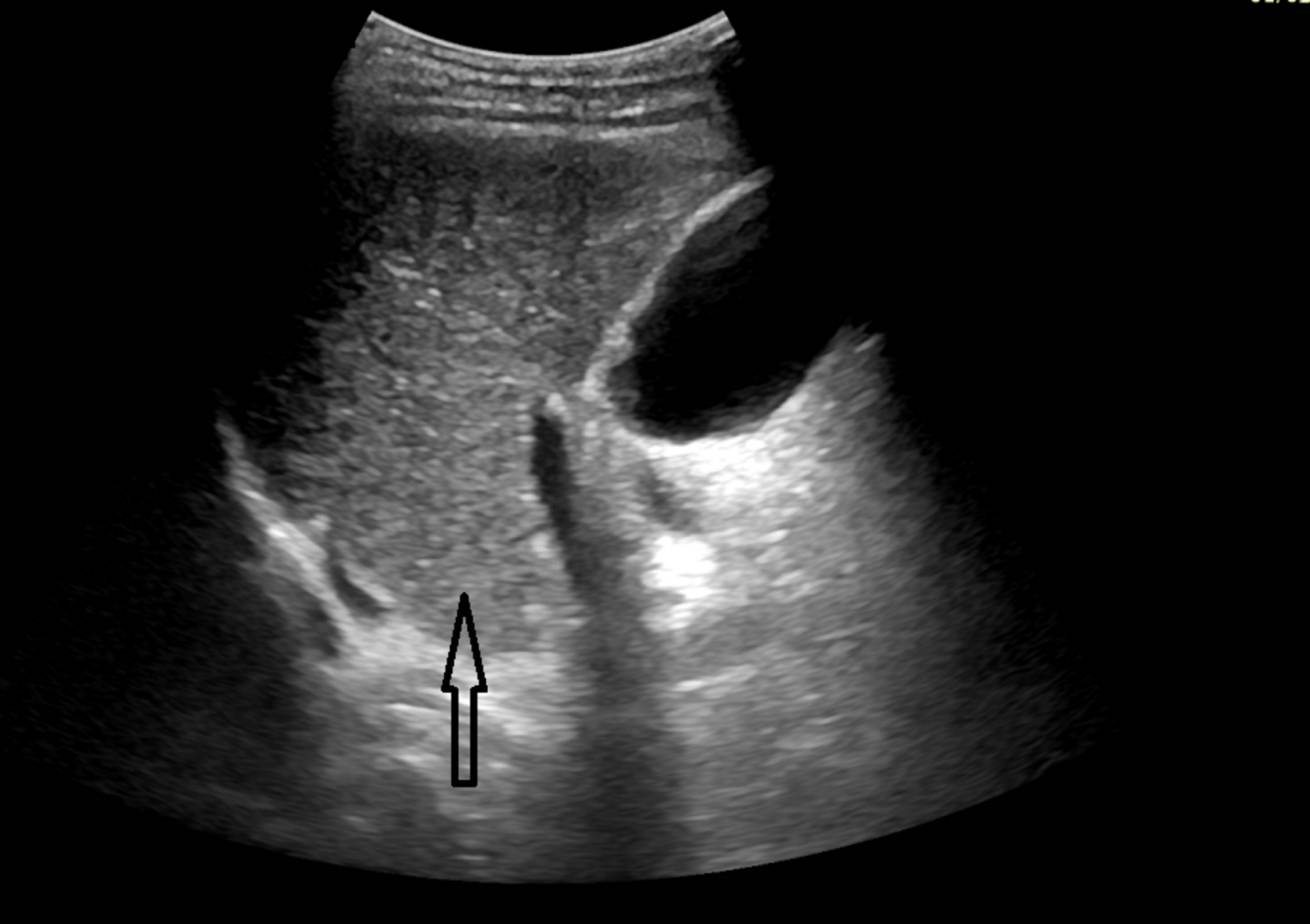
The arrow displays an ultrasound section through the right oblique parasaggital plane of liver demonstrating slightly coarse and heterogeneous liver parenchyma.
